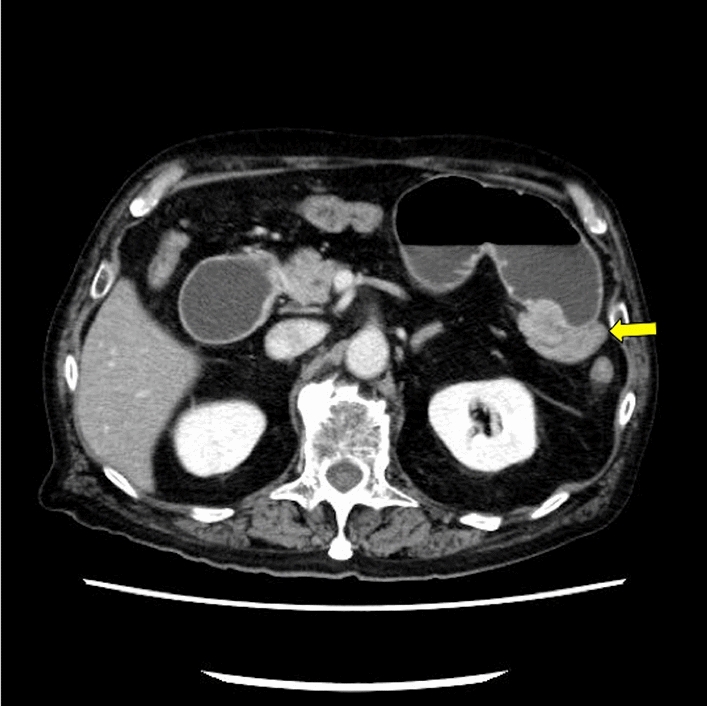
Contrast enhanced computed tomography revealed a 25 mm high-density mass in the jejunum (yellow arrow) with proximal bowel dilation
